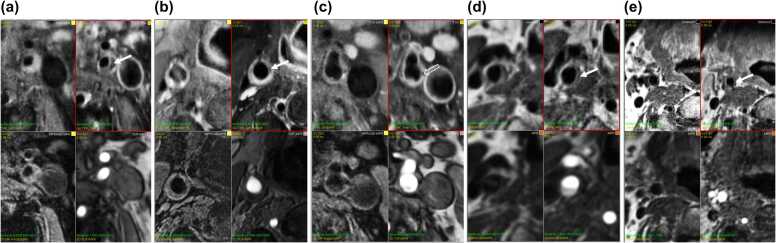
Example images in (a) a 61-year-old male acquired at site 1 with a dedicated carotid coil, (b) a 59-year-old female acquired at site 2 with a dedicated carotid coil, (c) a 55-year-old male acquired at site 1 with a dedicated carotid coil, (d) a 57-year-old male acquired at site 4 with a standard neck coil, and (e) a 57-year-old female acquired at site 4 with a standard neck coil. All images are axial and oriented perpendicular to the carotid artery. For each subject, the four carotid images are native T1-weighted (upper left), post-contrast T1-weighted (upper right), MP-RAGE (lower left), and TOF (lower right). Solid arrow: lipid-rich necrotic core. Open arrow: calcification. All scans were performed at 3T. Sites 1 and 4 used gadobutrol (Gadovist, Bayer Healthcare, Leverkusen, Germany), and site 2 used gadoterate (Dotarem, Guerbet, Villepinte, France), each administered at 0.2 mmol/kg body weight. Full imaging parameters, including post‑contrast timing and imaging planes, are detailed in Supplemental Table S1. *MP-RAGE* magnetization-prepared rapid acquisition gradient echo, *TOF* time-of-flight
